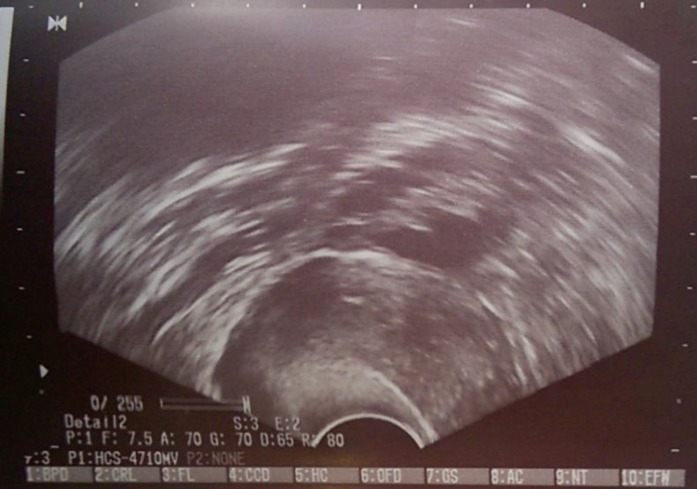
Sonography before induction of ovulation-normal ovary without dominant foliculs.
